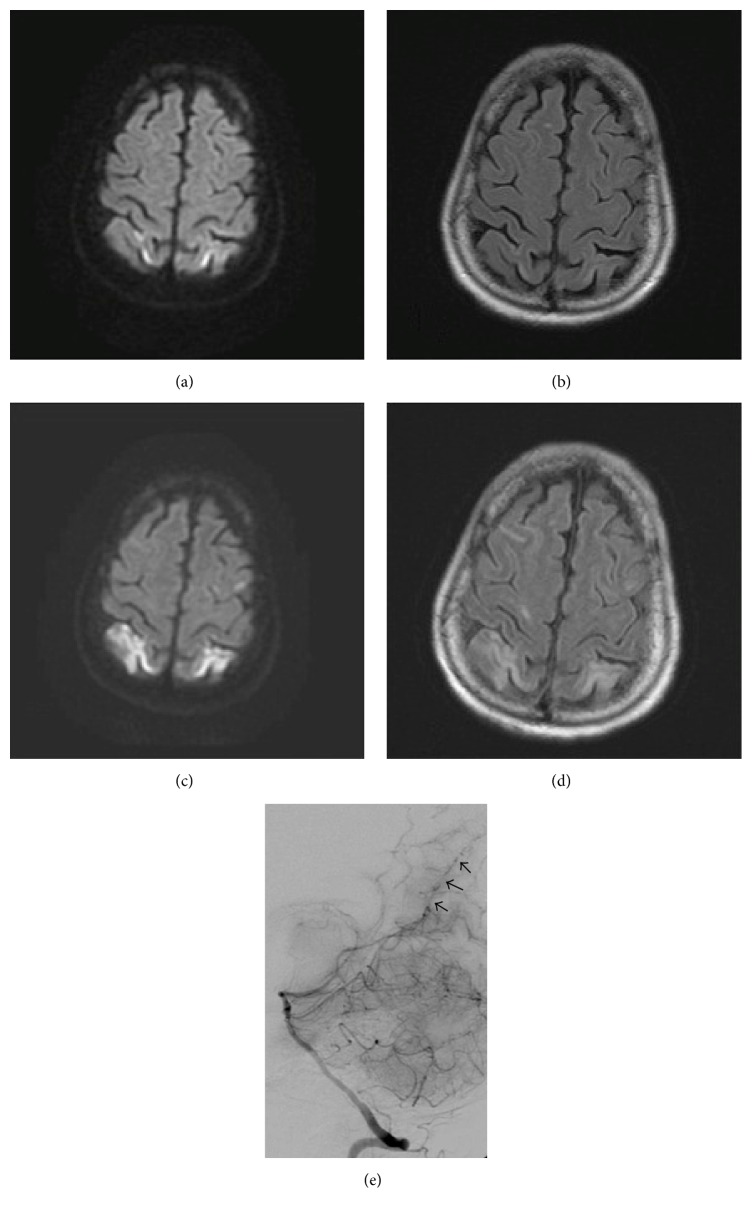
Relevant brain and blood vessel imaging highlighting vasculitic cause of multifocal strokes. MRI on day of admission with diffusion scan (a) significant for curvilinear increased signal in the cortical surface of both anterior parietal lobes extending into the postcentral gyrus on left, with additional foci of restricted diffusion within the periventricular white matter in both hemispheres (left > right). Subtle signal abnormalities are seen along cortical surface primarily in both anterior parietal lobes on FLAIR imaging (b). MRI performed 2 days after initial imaging showed progression of FLAIR signal (d) abnormalities with reduced diffusion (c) consistent with multifocal cortical infarcts involving the posterior frontal, occipital, left temporal, left frontal, and deep bilateral white matter. Cerebral angiogram (e) showing multifocal distal small vessel moderate to severe vasculitis predominantly involving the posterior circulation. Arrows point to areas of vessel beading and irregularity.
